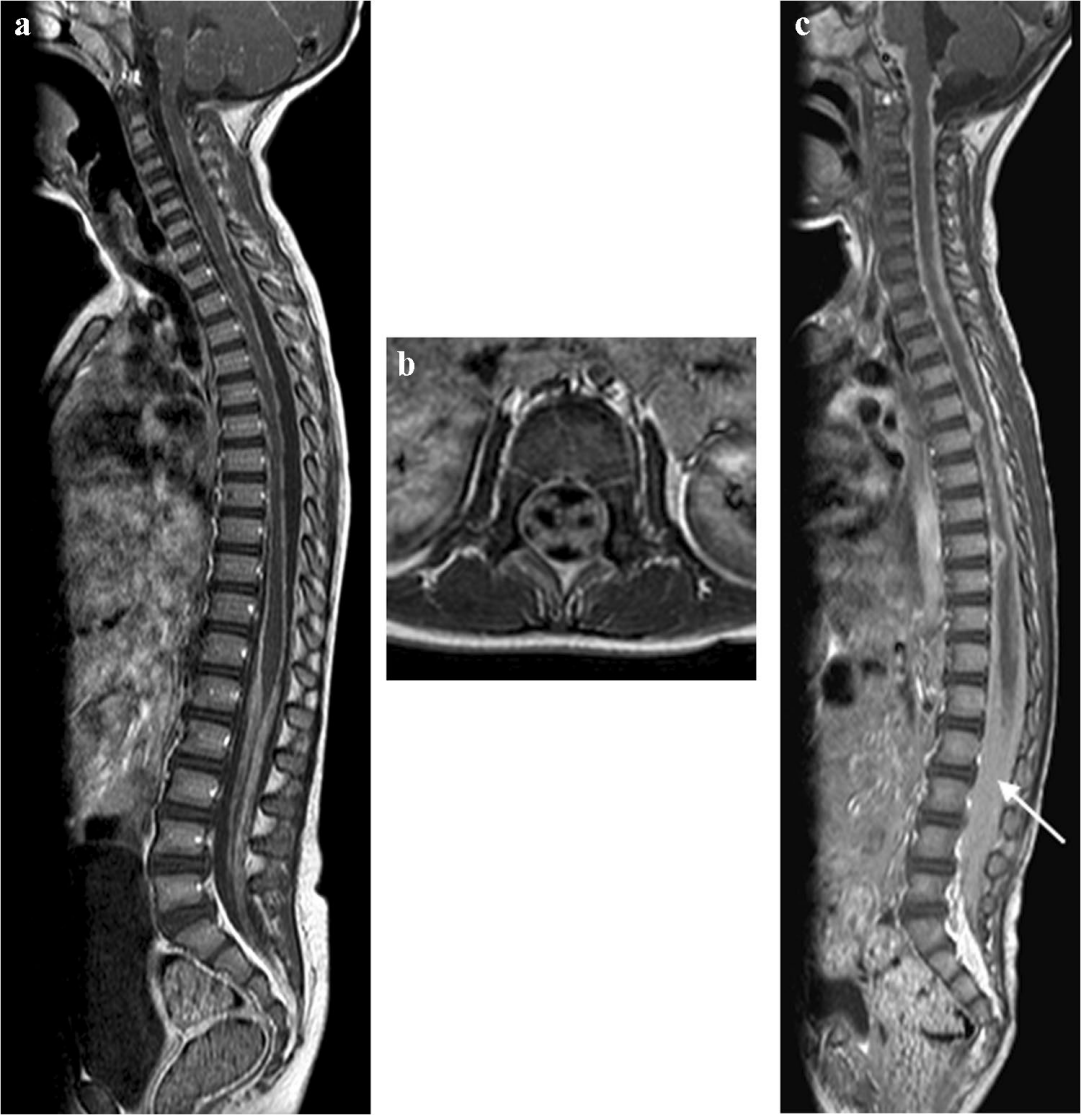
Sagittal (a) and axial T1 post-gadolinium (b) magnetic resonance imaging (MRI) in a 6-year-old girl with tuberculous (TB) meningitis show enhancement of the arachnoid with enhancement and clumping of the nerve roots. c Sagittal T1 post-contrast MRI in a different patient with TB meningitis, a 4-year and-10-month old girl who had repeated failed lumbar punctures, shows the spinal canal to be completely ocupied by enhancing tissue (*arrow*)
